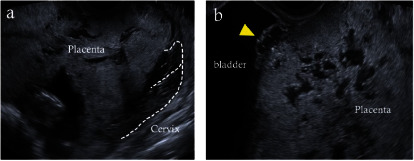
Ultrasound findings at 30 weeks of gestation. (a) Placental distension at the anterior uterine wall with a posterior deviation of the cervix. (b) Enlarged vascular protrusion (yellow triangle) and increased lacunae.
